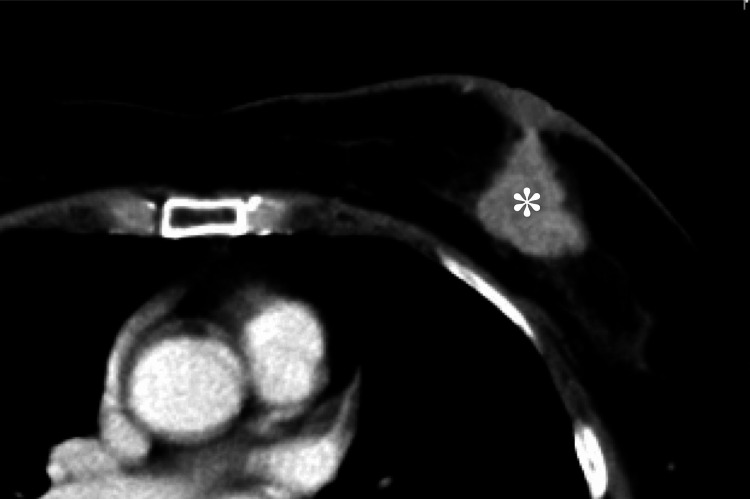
A chest CT image prior to NAC An irregular nodule is seen in the left breast (asterisk), indicative of breast cancer. Intraductal spreading towards the nipple is suspected. NAC: Neoadjuvant chemotherapy
